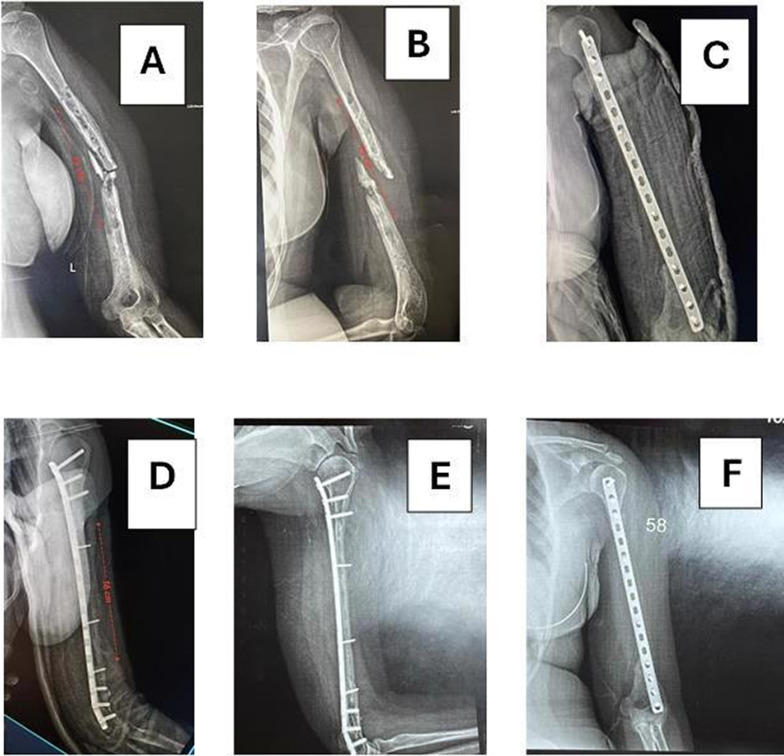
A, B Preoperative x ray before 1st stage, C, D immediate postoperative x ray, E, F last follow up x-ray after 1 year
